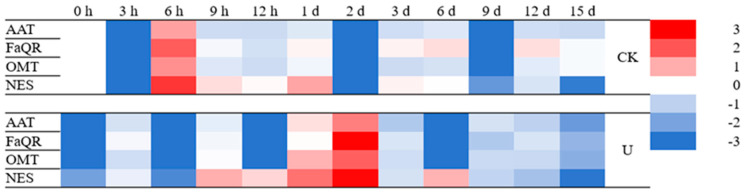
The relative expression of genes related to volatile synthesis of strawberry fruits from the control and ultrasound treatment.
